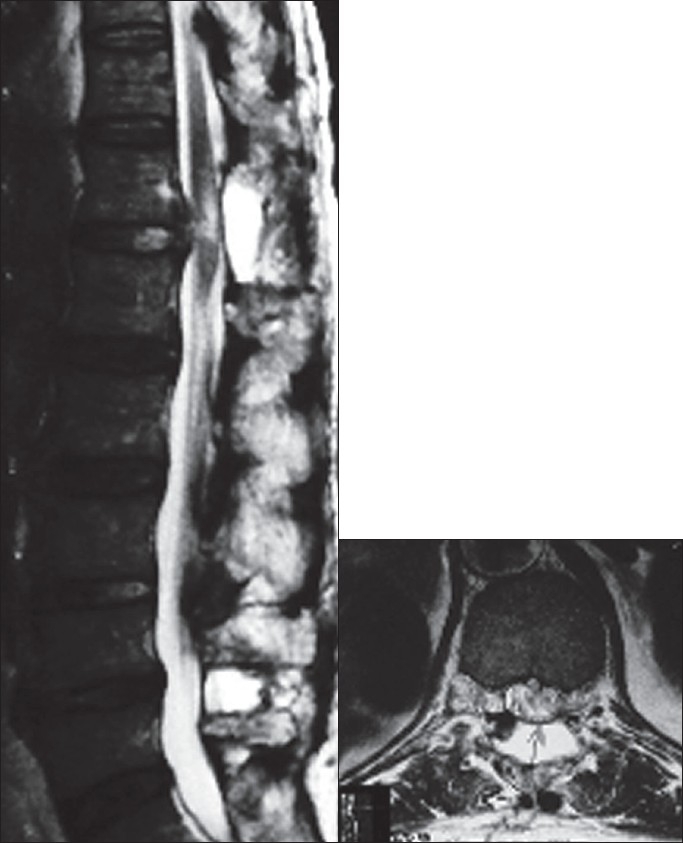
T2W MRI sagittal and axial images at 1 year followup showing expansion of cord and myelomalacia
